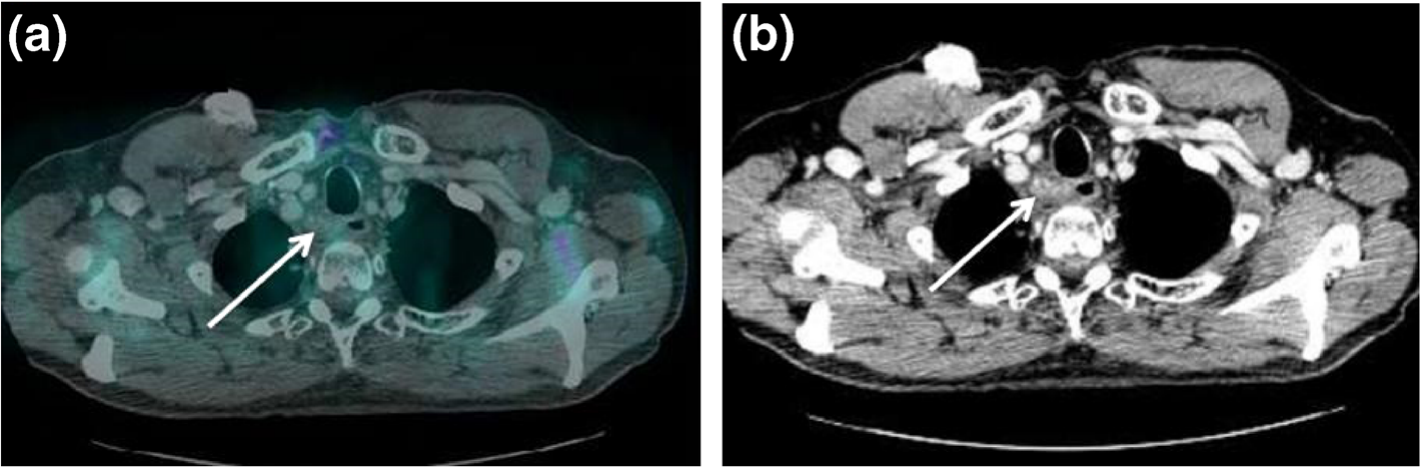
A 65-year-old male patient affected by pharyngeal cancer with lymph nodal relapse (secondary disease relapse: the first relapse in the neck lymph nodes had been treated with surgery and radiotherapy one year before). (a) Axial, contrast-enhanced, full-dose CT shows a small lymph node, which seems to infiltrate the upper esophagus. (b) As evident from contrast-enhanced PET/CT fused images, this lymph node does not display increased FDG uptake. Subsequent endoesophageal ultrasound biopsy confirmed the presence of lymph node metastasis. The patient was then submitted to chemotherapy. CT, computed tomography; PET, positron emission tomography; FDG, [^18^ F] fluorodeoxyglucose.
